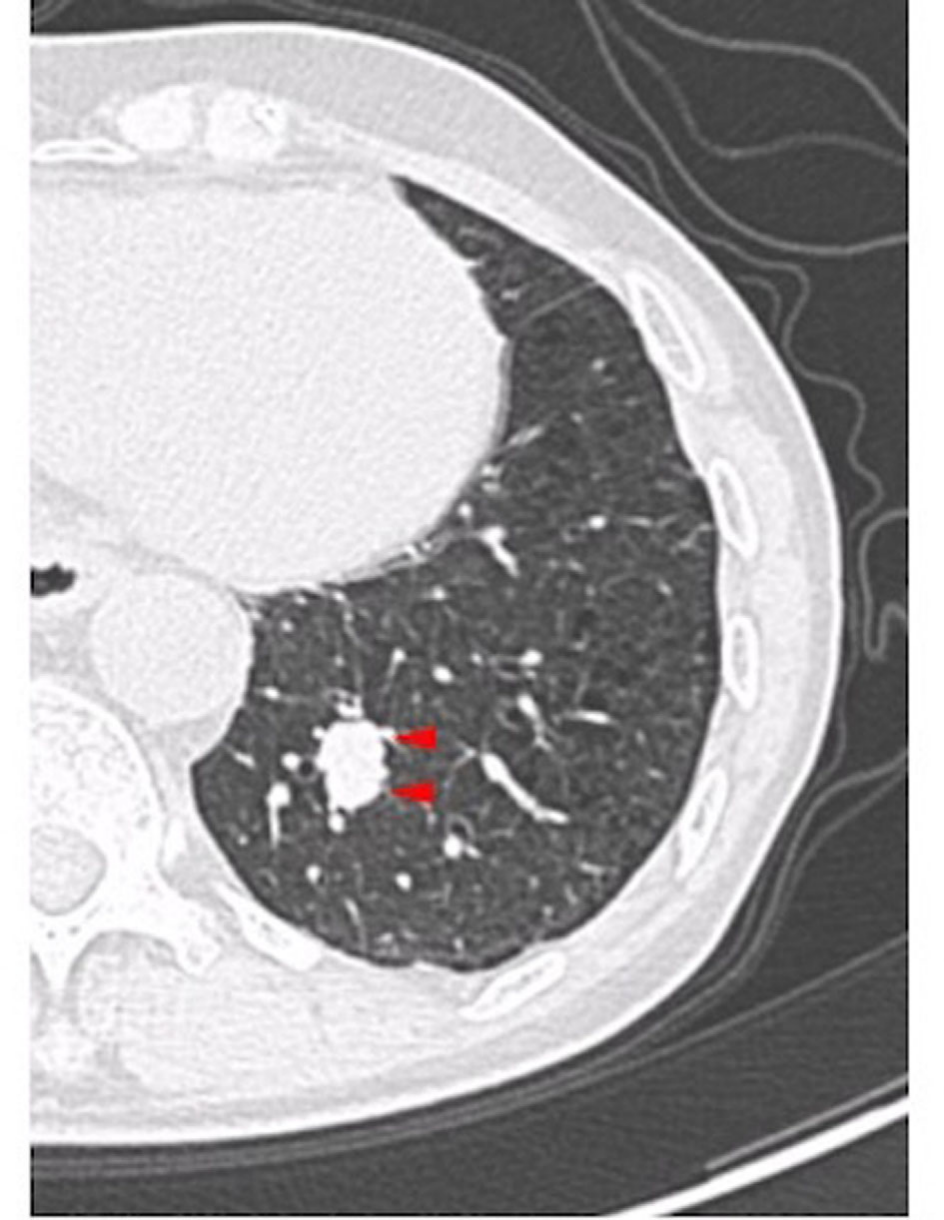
Preoperative computed tomography (CT) revealed a 20‐mm diameter solid nodular shadow (arrowheads) in the posterior basal (S10) segment, which was strongly suspected to be a pulmonary metastasis from colorectal cancer
